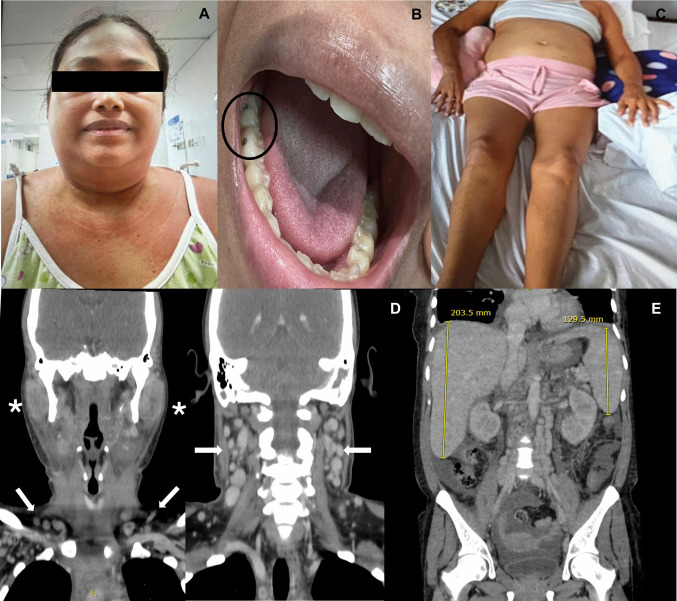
A Bilateral enlargement of the parotid glands. B Dental caries in atypical distribution areas (circle). C Anasarca. D Contrast-enhanced CT scan of the head and neck showing bilateral parotid gland enlargement (asterisk) and lymph nodes larger than 2 cm in the submandibular, cervical, and supraclavicular chains (arrows). E Contrast-enhanced CT scan of the abdomen revealing hepatosplenomegaly
